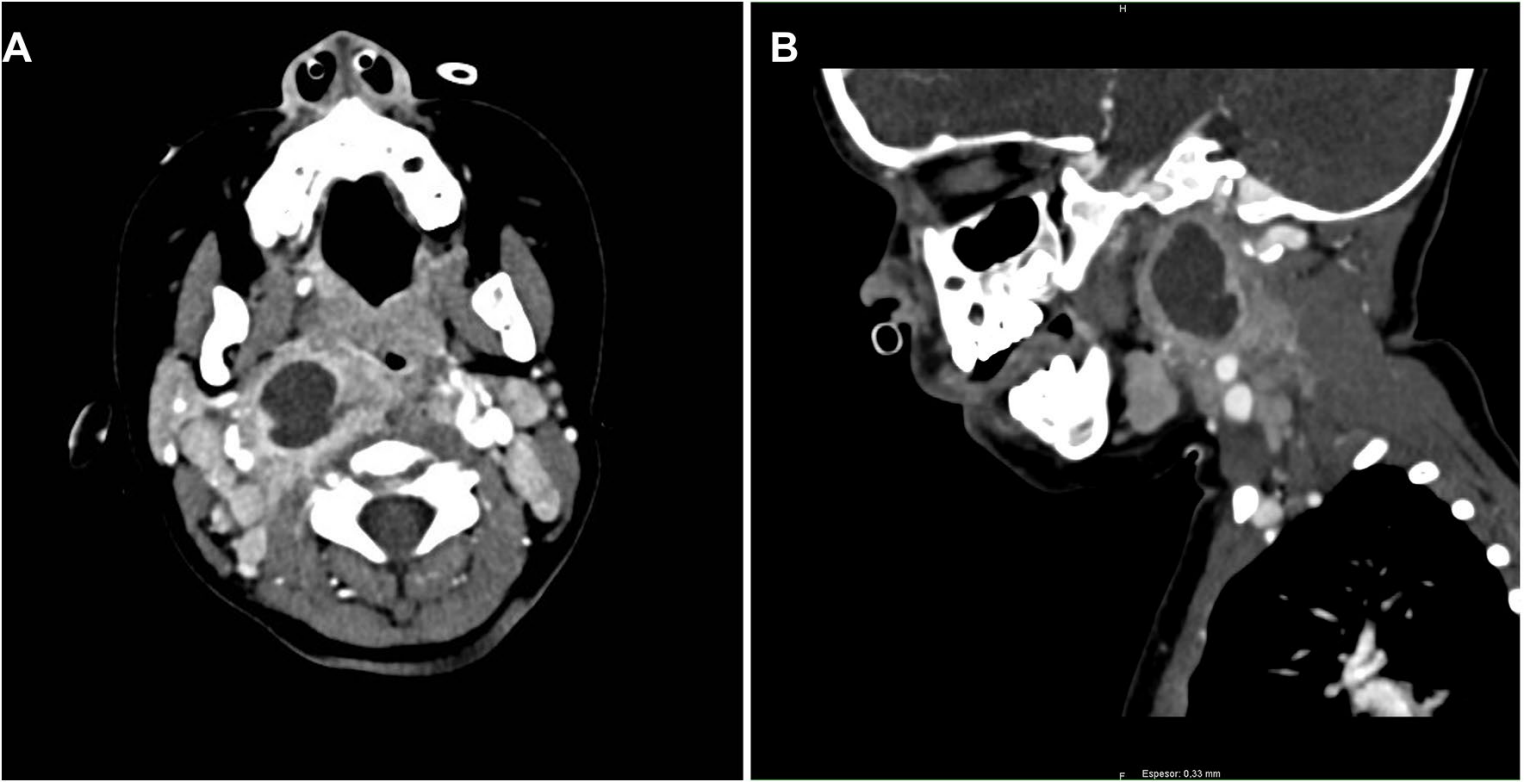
A Axial contrast enhanced CT scan of a 2-year-old patient showing a sharply demarcated lucency (arrow) in the right PPS (19.6 × 17.3 mm). B Sagittal contrast enhanced CT scan of the same patient
